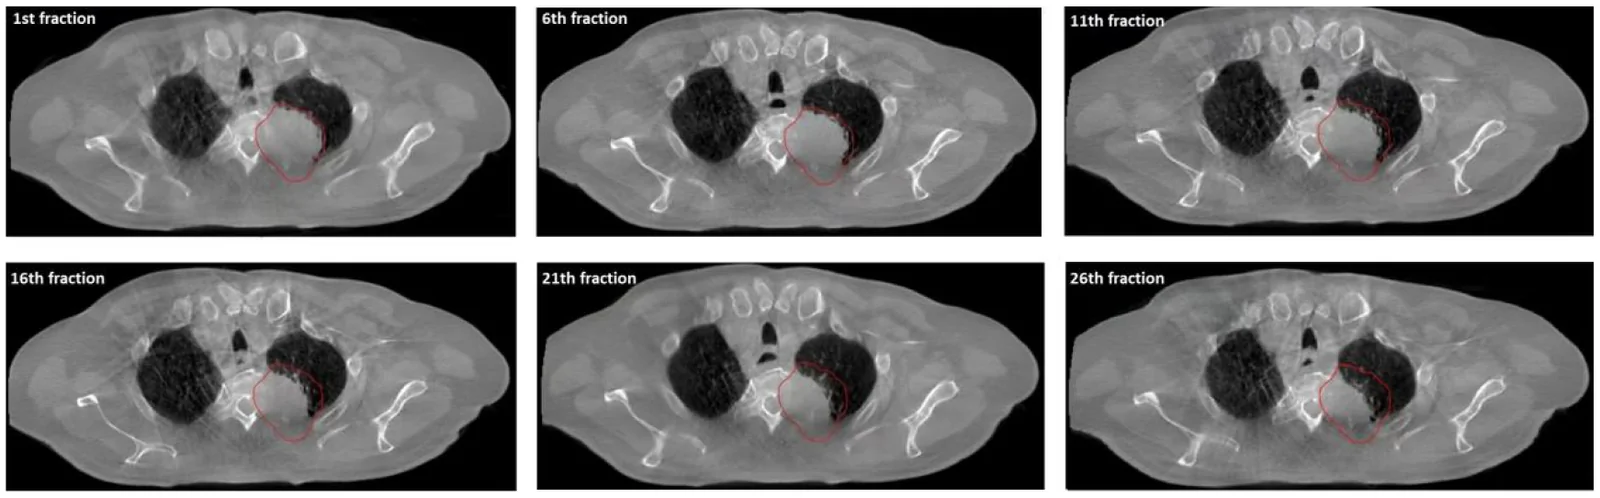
Dynamic changes of the primary tumor on the CBCT. The picture shows a gradual course of primary tumor shrinkage from baseline at 1^st^, 6, 11, 16, 21, and 26 fractions from the radiotherapy start. Redline shows the primary tumor at baseline.
